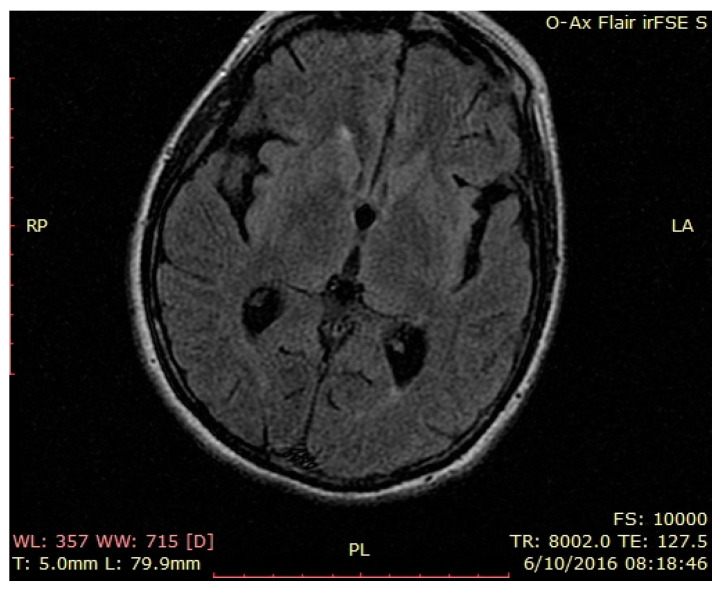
Second brain MRI, FLAIR images, axial insular image.
